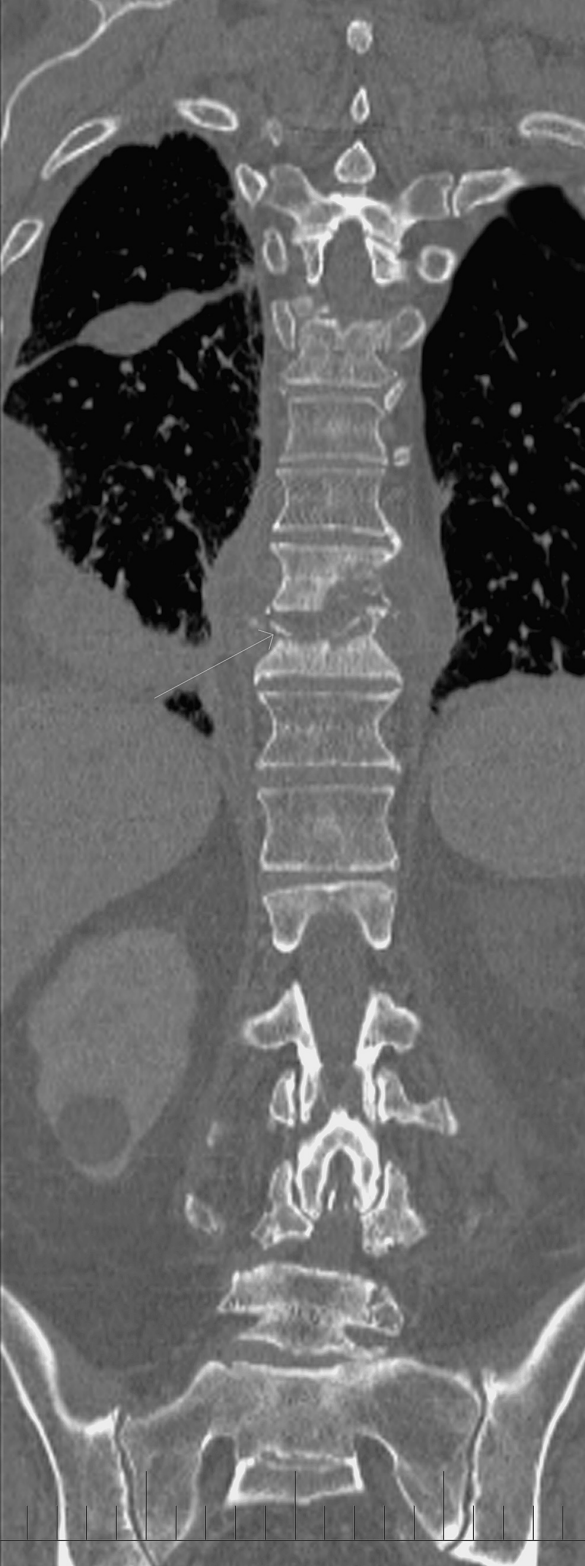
Computed tomography coronal view of the thoracic spine from the initial visit This demonstrates bony destruction of the T9 vertebral body.
